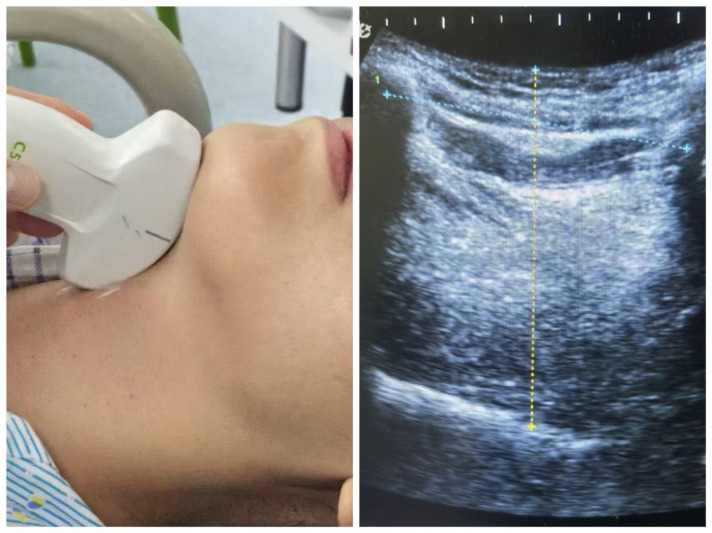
The method of tongue ultrasound measurement. (A) The low-frequency convex-array probe was placed to the skin of the neck in the midline in the submental sagittal plane; (B) The probe was adjusted to obtain entire tongue contour and the hyoid bone clearly on the screen. The TT of this patient is 62.4 mm and the hyomental distance is 53.1 mm as shown in the figure.
